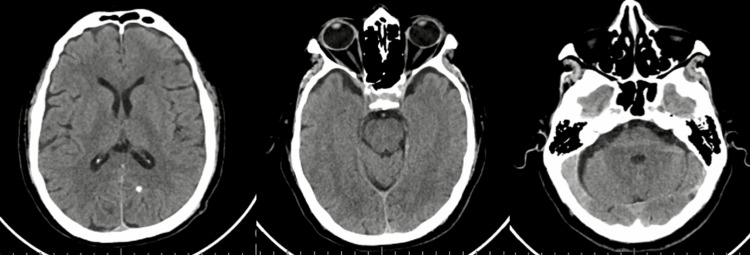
Axial views of the head CT scan were normal
